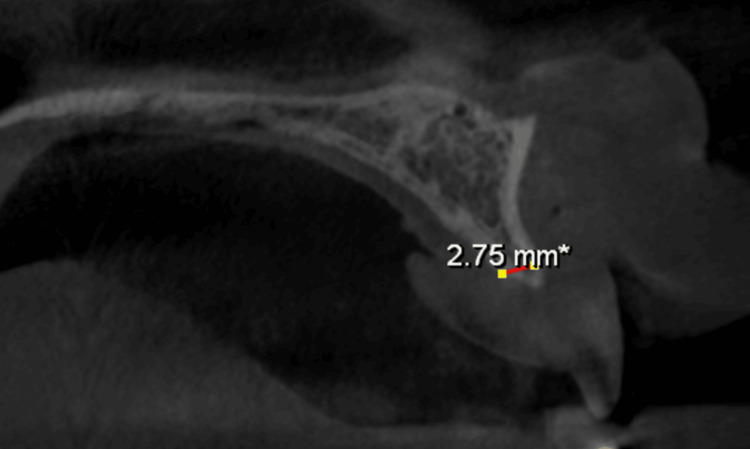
CBCT (sagittal view) indicating a preoperative maxillary ridge width of 2.75 mm. CBCT, cone-beam computed tomography
